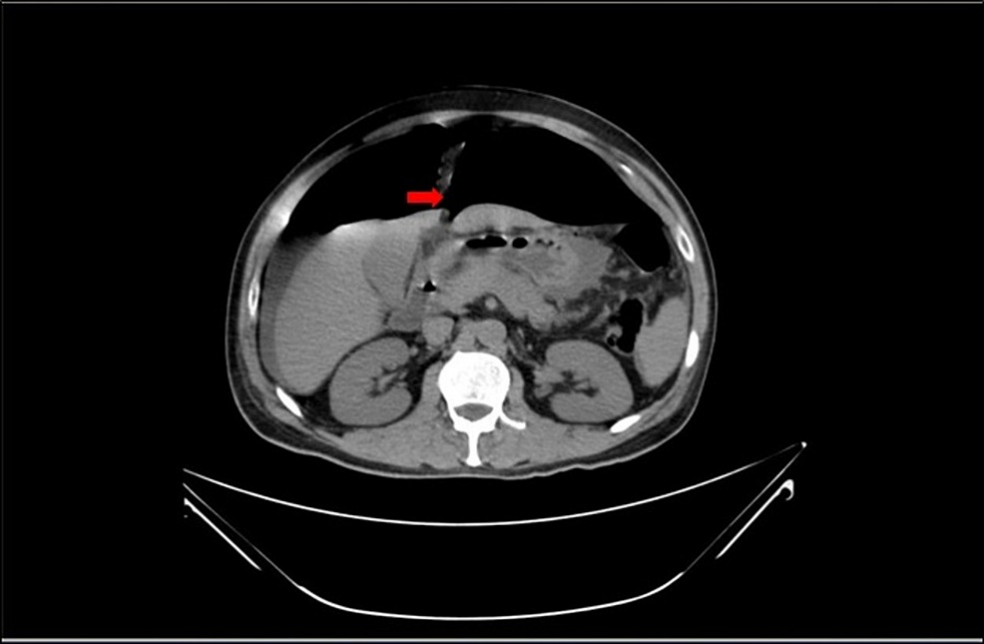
CT abdomen - transverse view showing falciform ligament sign.
